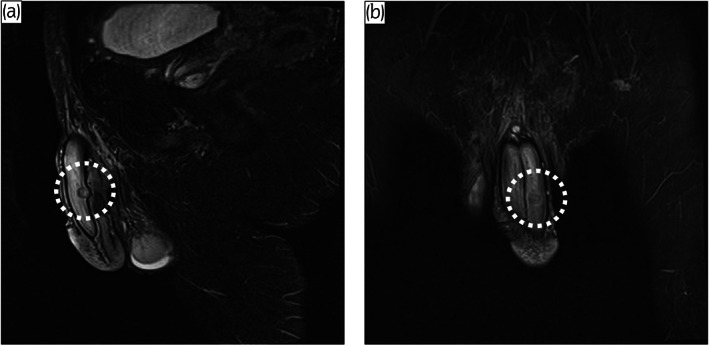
T2‐weighted MRI sagittal (a) and coronal (b) section. The circle shows inhomogeneous low signal intensity.
